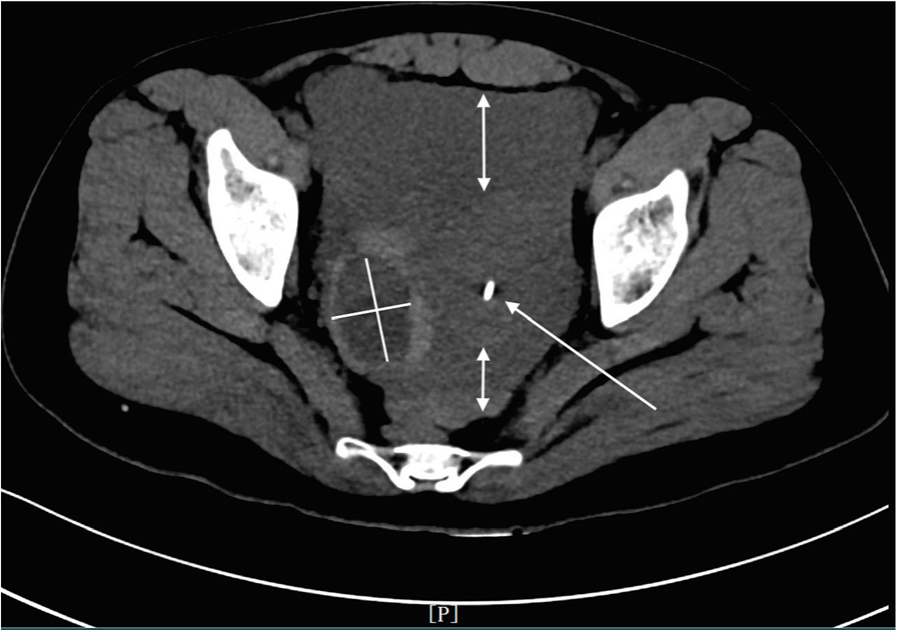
CT findings of ROCLC. CT image of a post-coital patient who presented with persistent abdominal pain for a period of half a day; HCG test was negative. The crosshair indicates the maximum and minimum diameter of the ruptured corpus luteal cyst; the double-headed arrows indicate the depth of pelvic fluid; the long arrow indicates the location of the uterus and IUD
